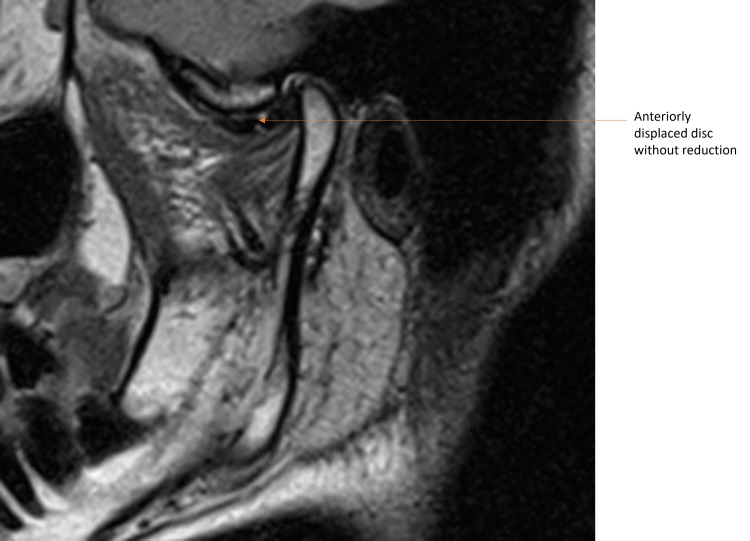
MRI scan (sagittal section) showing non-reducing anteriorly displaced disc during closing of the mouth
